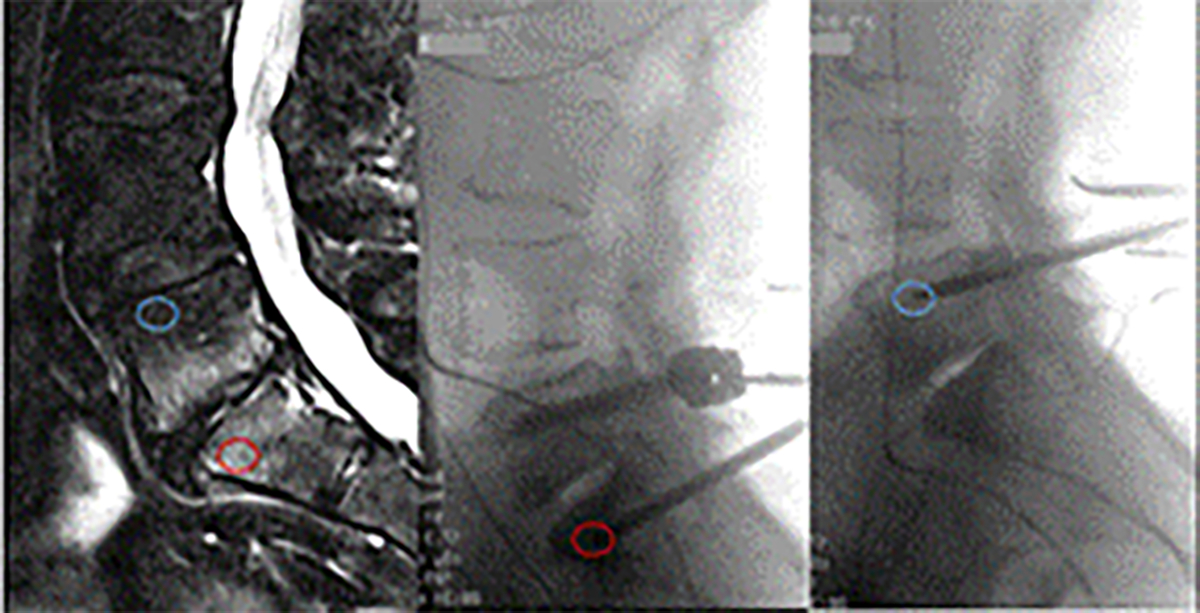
MC1 at L5/S1. Pre-operative sagittal T2w MRI (left), intraoperative sagittal X-ray during harvesting of MC1 aspirate (middle) and intra-patient control aspirate (right). Red and blue circle indicate location of MC1 and control aspirate, respectively.
